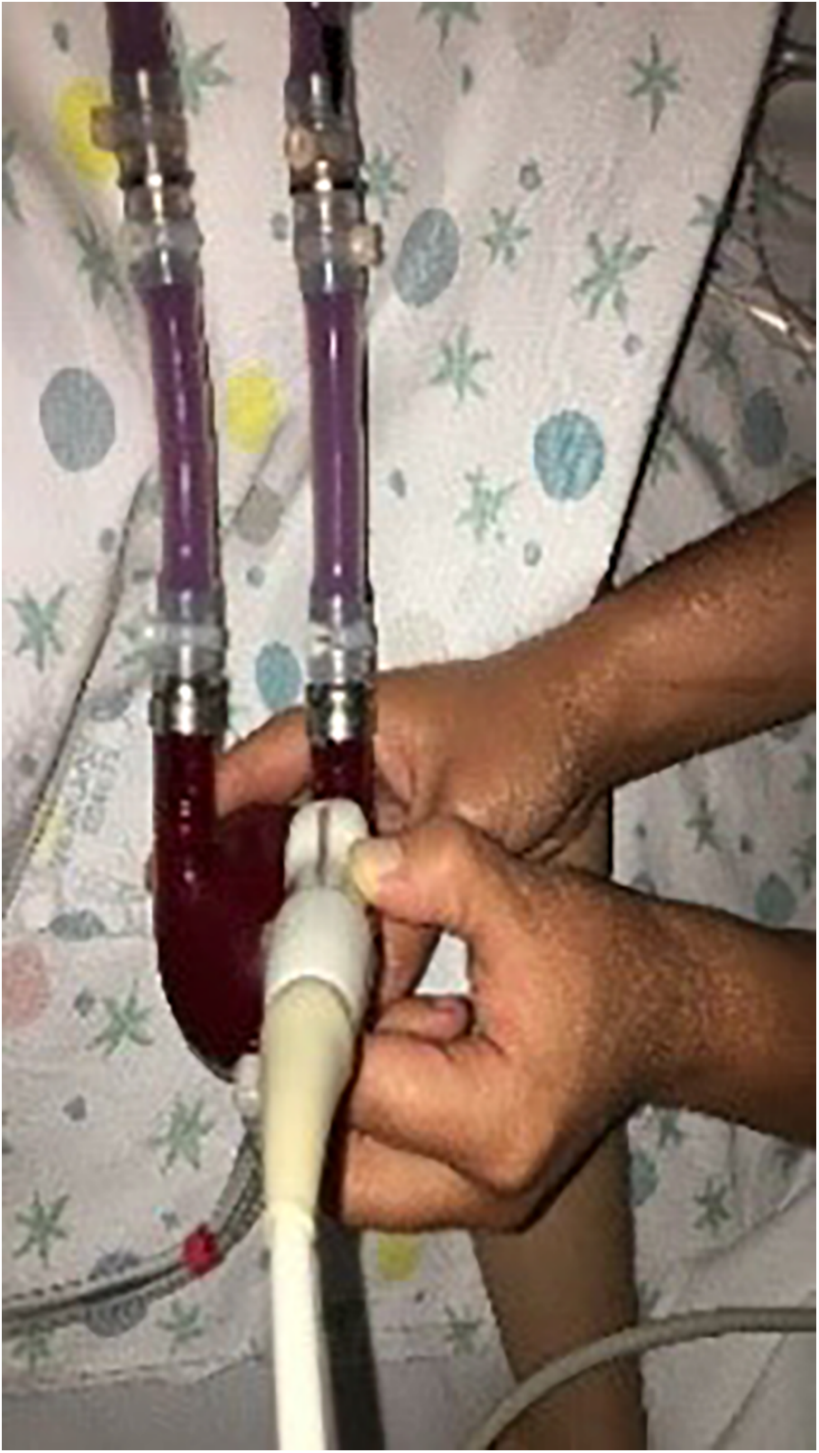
Echocardiographic evaluation by direct positioning of the transducer on BH components (inflow & outflow valves and membrane).
